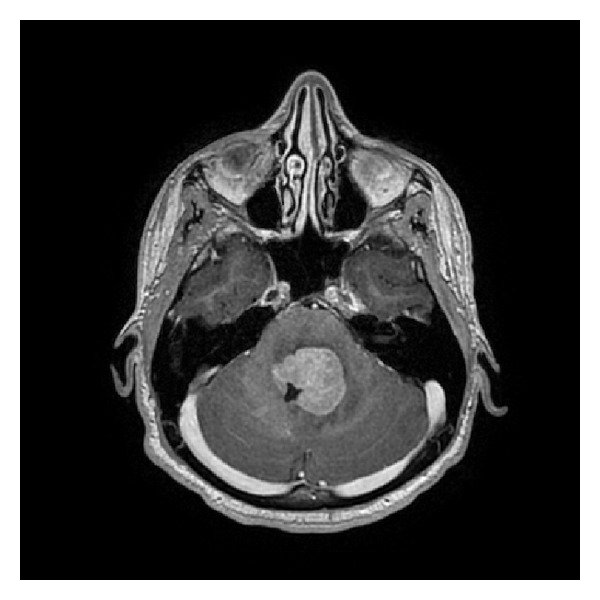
T1-weighted, gadolinium-enhanced axial magnetic resonance image, showing a well-demarcated intensely enhancing subependymal lesion occluding the lumen of the fourth ventricle and causing moderate peritumoral edema.
